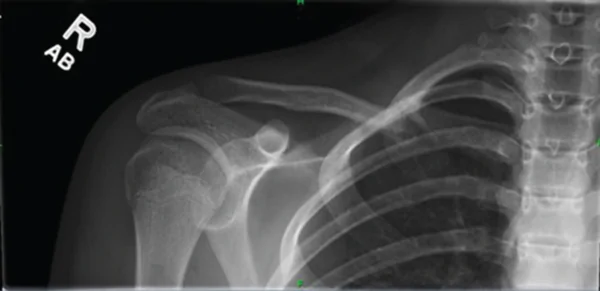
Day 50 radiographs demonstrating mild interval separation at the fracture site following reported premature backpack use. Differences in radiographic projection, positioning, and magnification cannot be excluded as contributing to the apparent interval change.
